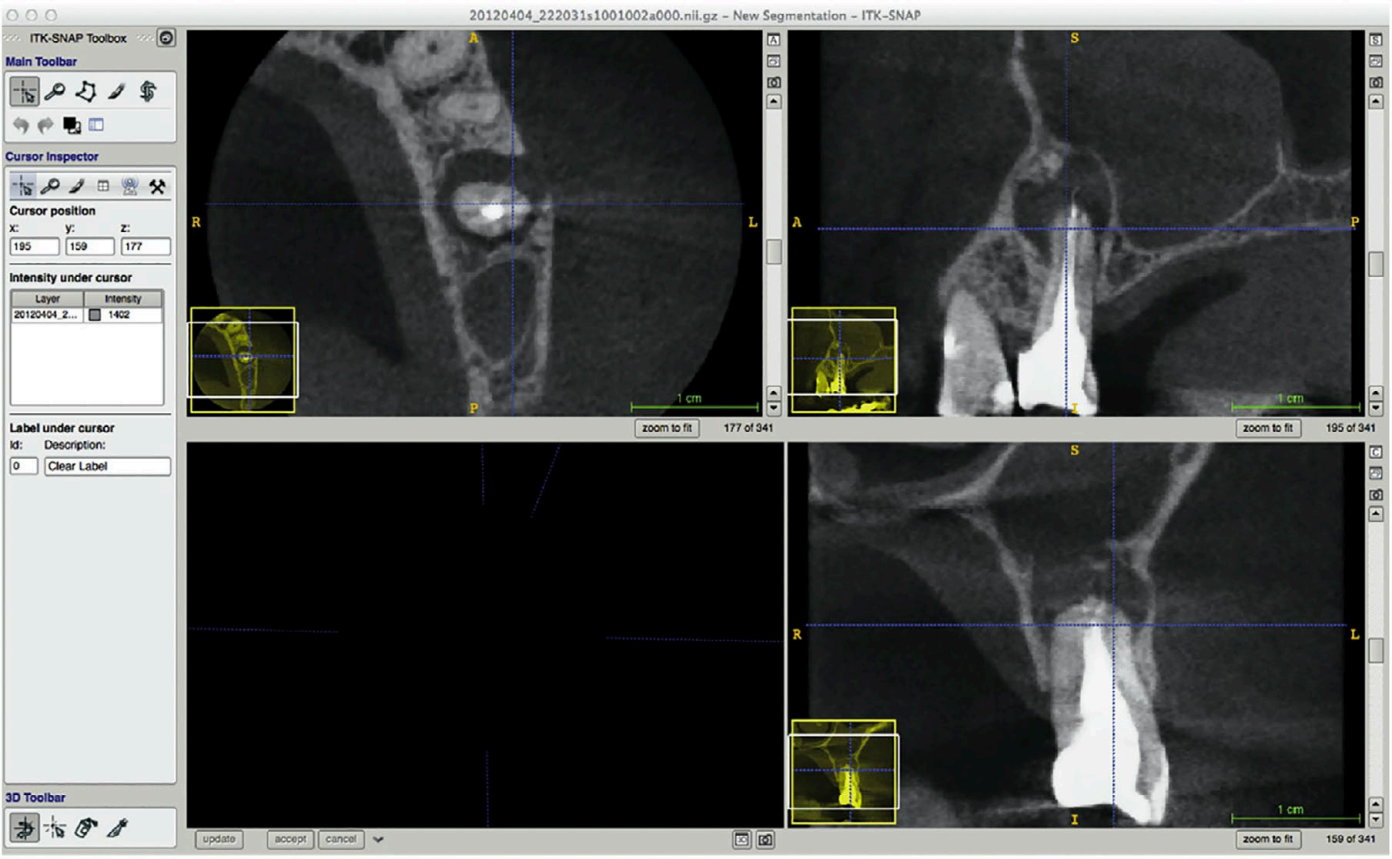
Item 11h – Deidentified CBCT images. Reprinted from *Journal of Endodontics*, Vol 43, Schloss, T., Sonntag, D., Kohli, M. R., & Setzer, F. C. A Comparison of 2‐ and 3‐dimensional Healing Assessment after Endodontic Surgery Using Cone‐beam Computed Tomographic Volumes or Periapical Radiographs, Copyright (2017) with permission from Elsevier.
